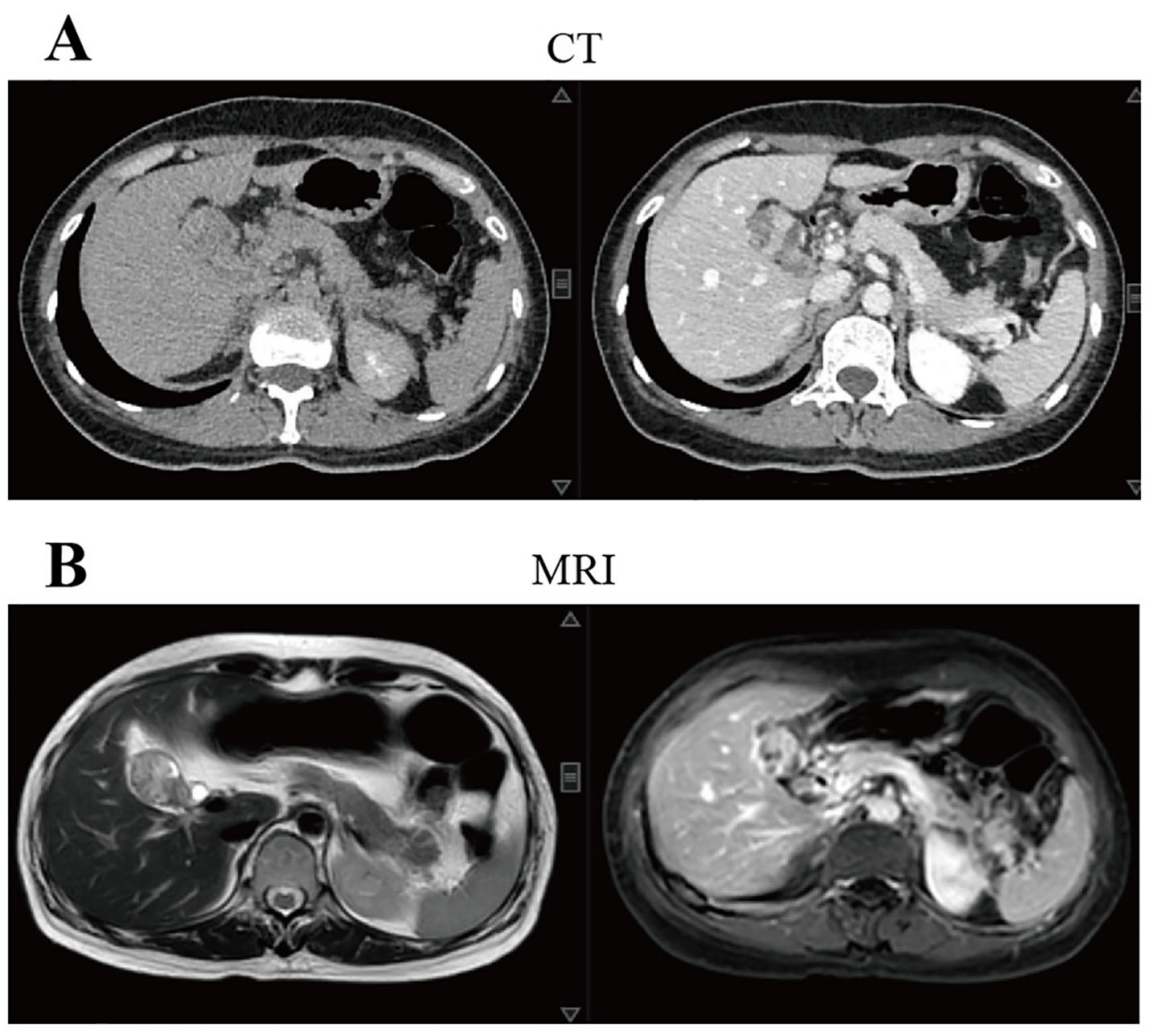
Imaging tests at the time of the patient’s first visit to our hospital suggested that gallbladder cancer was possible. (A) CT showed that the wall of the gallbladder was thickened and a mass measuring 3.6 cm×2.5 cm at the largest level was formed, with uneven enhancement after enhancement. The walls of the right and left intrahepatic bile ducts were slightly thicker and the lumen is slightly dilated; (B) MRI showed thickening of the wall of the gallbladder and formation of a mass with a maximum dimension of about 3.4 cm×2.2 cm, which was considered malignant in nature and was adjacent to the liver. The intrahepatic bile ducts and common hepatic duct were slightly dilated and the wall was slightly thick. CT, computed tomography; MRI, magnetic resonance imaging.
